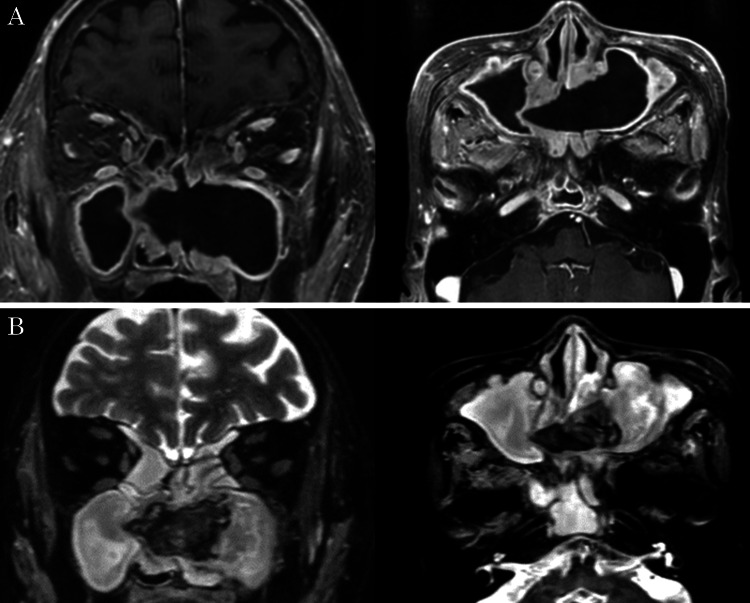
Gadolinium-enhanced sinus MRI (A) T1-weighted and (B) T2-weighted images show a mass lesion with low-intensity signals in the left maxillary sinus extending to the contralateral maxillary sinus through the nasal septum MRI: magnetic resonance image
